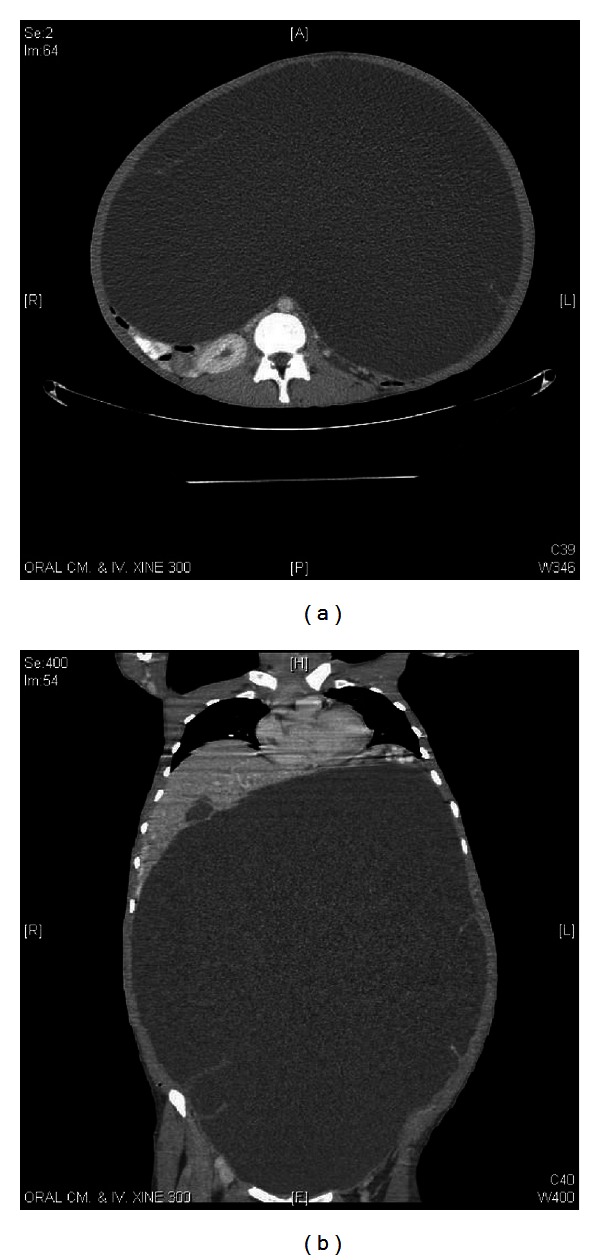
Axial and coronal computerized tomography (CT) scan images showing a huge ovarian cyst of patient 4.
